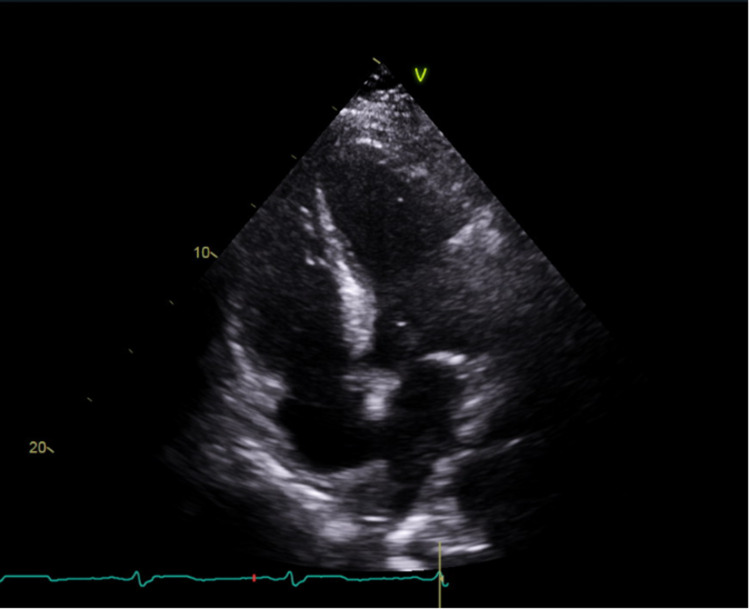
Transthoracic echocardiogram showing a four-chamber view, with no wall motion abnormalities seen.
